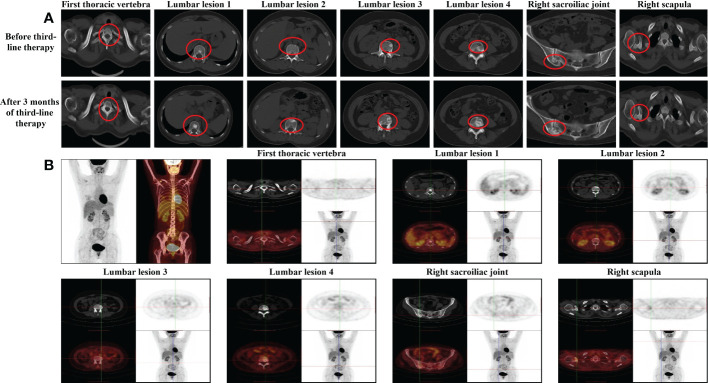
Imaging examinations during the third-line endocrine therapy (A) CT evaluation of the bone lesion after 3 months of third-line endocrme therapy in October 2021. (B) PET/CT evaluation of each bone lesion after 3 months of third-line endocrine therapy in October 2021.
